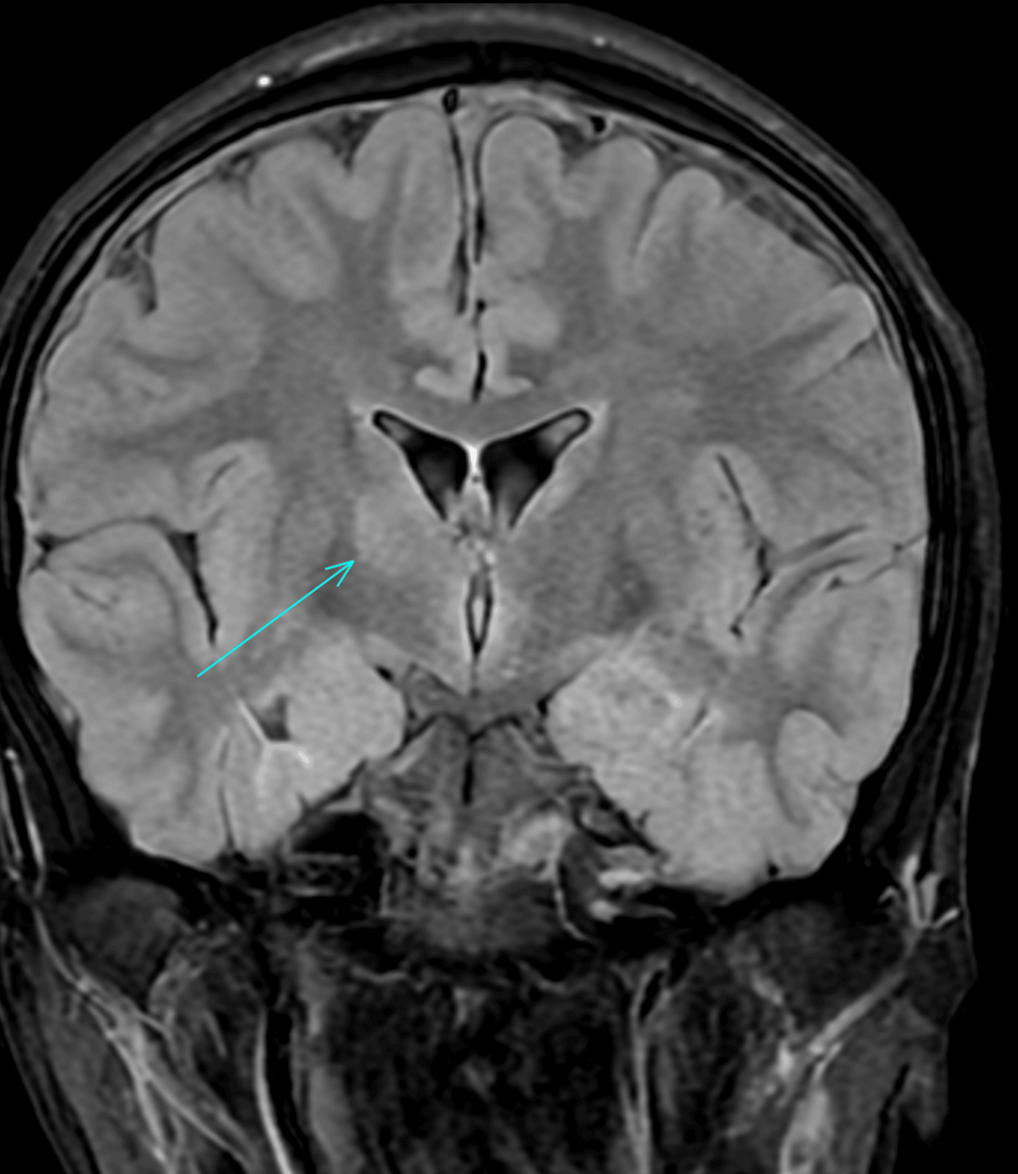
MRI head scan highlighting inflammatory changes (arrow, coronal view) MRI, magnetic resonance imaging
